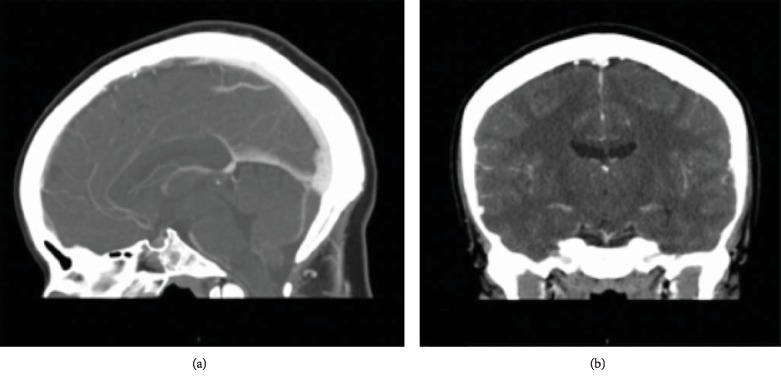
(a) CT venogram with sagittal images resolution of filling defects. (b) Coronal images with resolution of filling defects.
